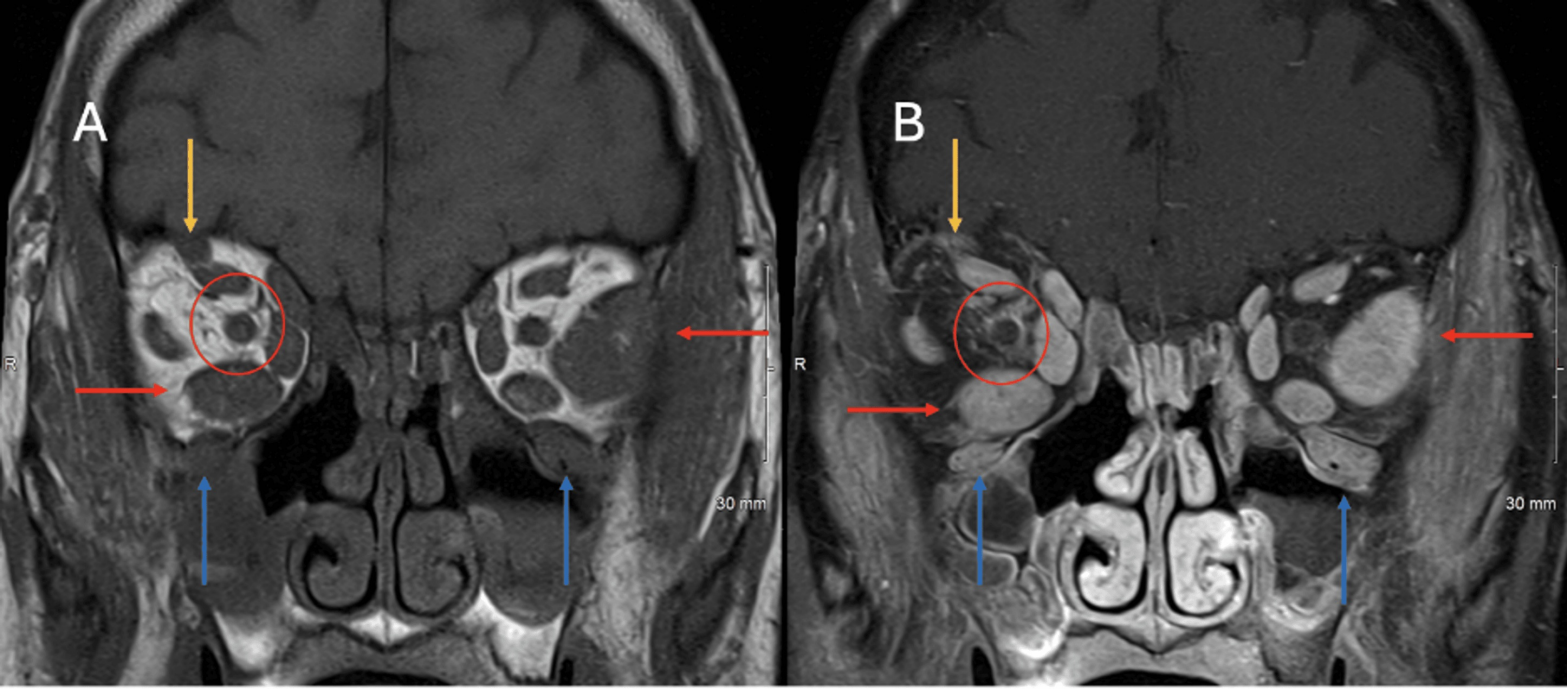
Coronal MRI scans of orbits with and without contrast Coronal T1 weighted MR image without contrast (A) and coronal fat-saturated T1 weighted MR image at the same level with contrast (B). There is thickening with enhancement of the supraorbital (orange arrow) and infraorbital (blue arrows) nerves. A red arrow (pointing to the left) and another red arrow (pointing to the right) demonstrate the inferior and lateral rectus muscles. There is an asymmetric enlargement of enhancing extraocular muscles, most notably the left lateral rectus. Peri-ophthalmitis about the right optic nerve sheath manifests as nerve sheath thickening with enhancement and adjacent fat stranding, identified to best advantage on the fat-saturated T1 post-contrast images. All findings are related to IgG4-RD. Incidental note made of post-operative changes of paranasal sinuses with inflammatory mucosal hypertrophy and mucosal retention cysts (likely not related to IgG4-RD). IgG4-RD - immunoglobulin G4-related disease
